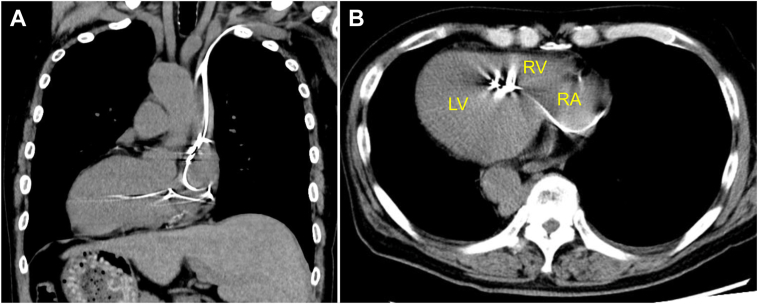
Computed tomography images showing atrial and ventricular leads after the operation. Coronal (A) and axial (B) computed tomography demonstrate the morphologic cardiac chambers in dextrocardia. Atrial and ventricular pacing leads are appropriately positioned within their corresponding morphologic chambers. LV = morphologic left ventricle; RA = morphologic right atrium; RV = morphologic right ventricle.
